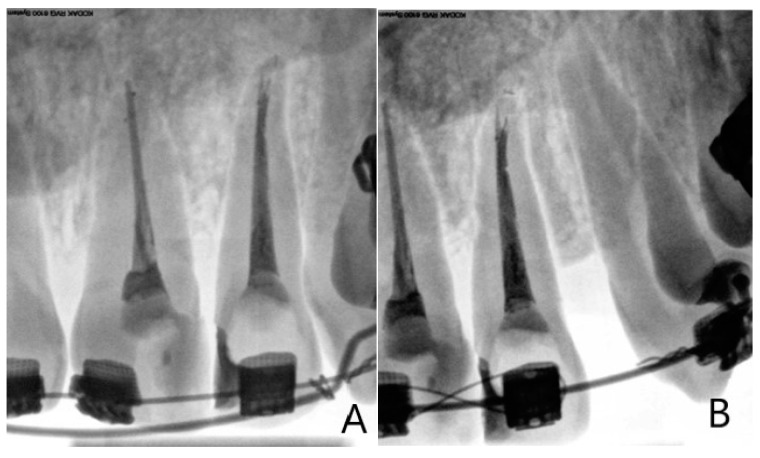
Intraoral periapical radiographs demonstrating the changes in periodontal ligament space width (Kodak software negative/positive mode to enhance subtle periodontal space changes. (A) Baseline intraoral periapical radiograph taken at the first appointment. (B) Intraoral periapical radiograph taken at the third appointment during treatment with FOAs. Evident widening of periodontal ligament space of distal/lateral aspect.
